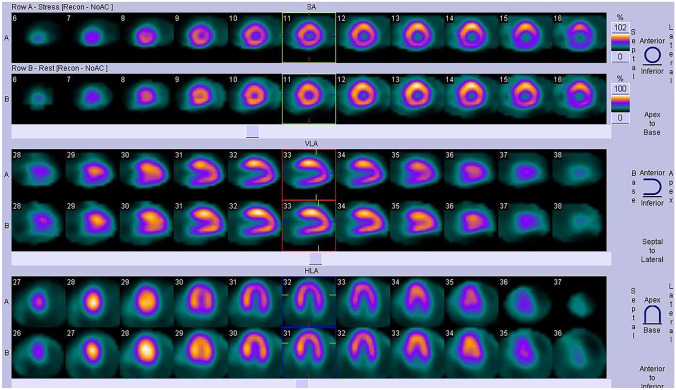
SPECT imaging: patient with asymmetric septal hypertrophy. Perfusion defects were detected only during exercise in the basal segments of septum and in the left ventricular inferolateral wall. Perfusion defects completely resolved at rest
